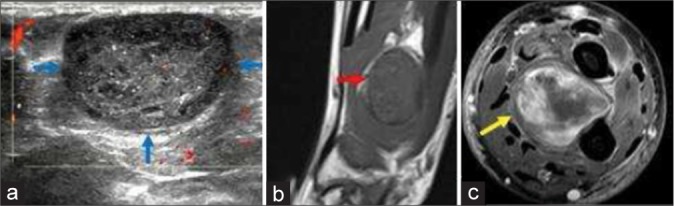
A 45-year-old woman with schwannoma who presented with swelling around wrist region. (a) Color Doppler ultrasound image showing a well-defined oval hypoechoic mass with smooth outline and hypoechoic rim. Note the trace internal vascularity within the mass (b) Non-contrast T1-weighted sagittal. Magnetic resonance images (MRI) image of the same patient showing well-defined hypointense mass with split-fat sign (red arrow). (c) Fat saturated axial T1-weighted contrast-enhanced MRI image shows heterogeneous enhancement of the mass (yellow arrow) with central necrosis.
